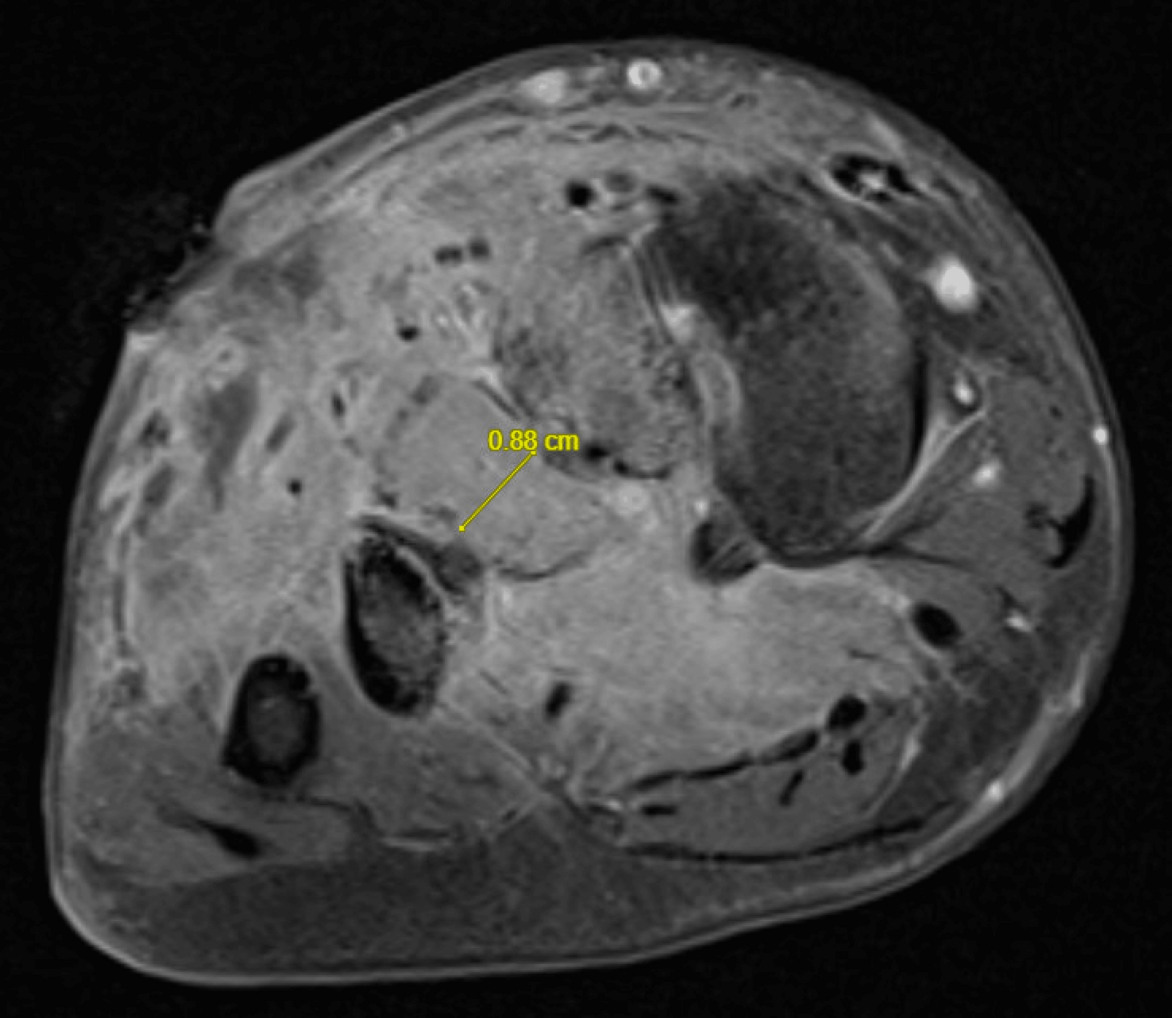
Repeat foot MRI revealed osteomyelitis of the third metatarsal with involvement of the adjacent second and fourth metatarsal bases, diffuse cellulitis, and multiple tiny foci of bone edema in the forefoot and midfoot. A 0.88 cm area of inflammation was noted, concerning for osteomyelitis of the third metatarsal with diffuse overlying cellulitis.
